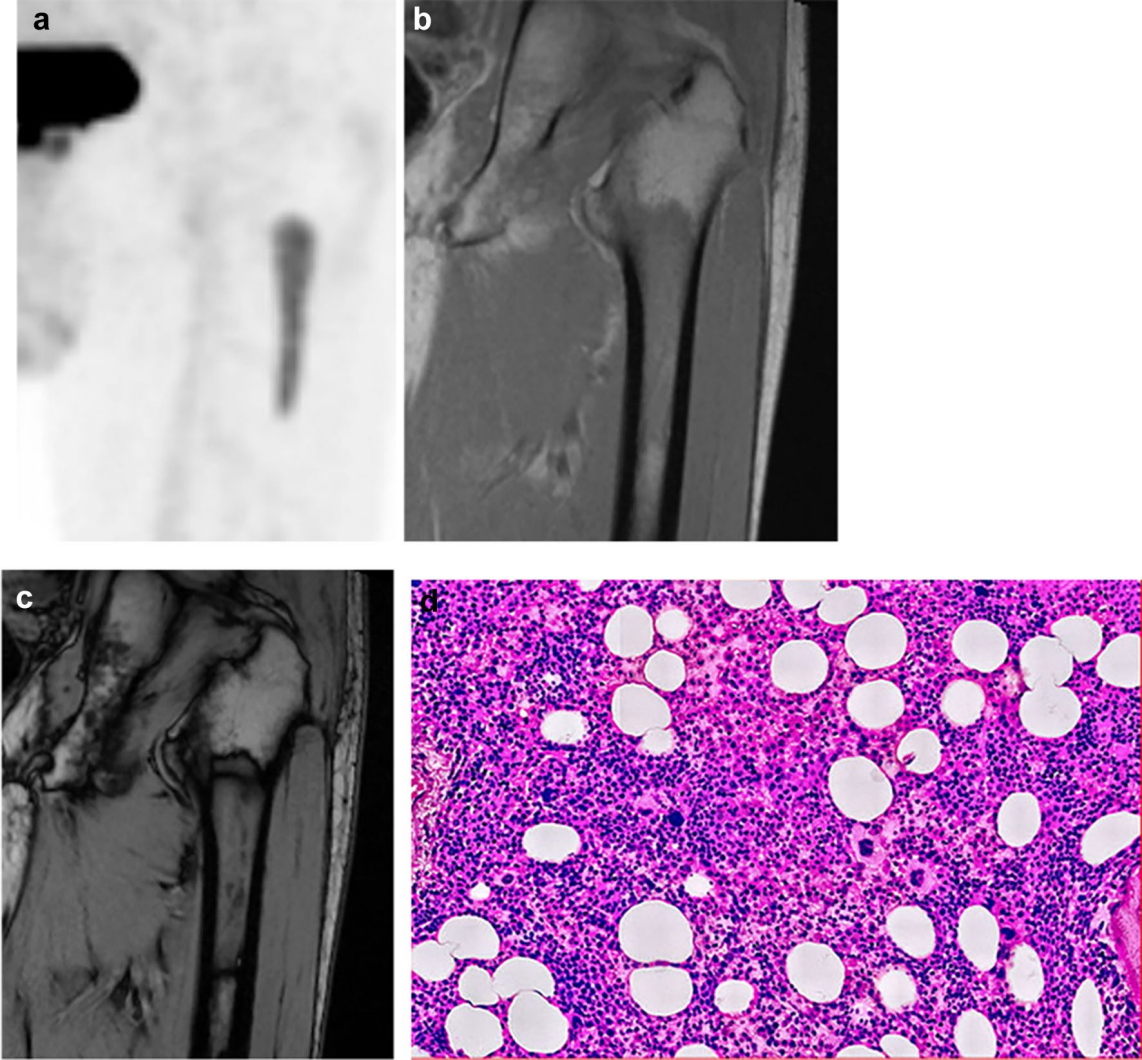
A 69-year-old male patient with no history of malignancy. Coronal FDG-PET CT image a shows a focal hot spot in the left femur. SUV_max_ of the left femur lesion is 4.0. Coronal in-phase b demonstrates a signal intensity of 175.1 whilst the opposed-phase c shows a signal intensity of 155.4. Signal change ratio (SCR) on CSI wa﻿s − 11.2%, compatible with a benign lesion. Biopsy was performed to exclude the possibility of malignant lesion, which revealed hyperplastic hematopoietic bone marrow (d)
